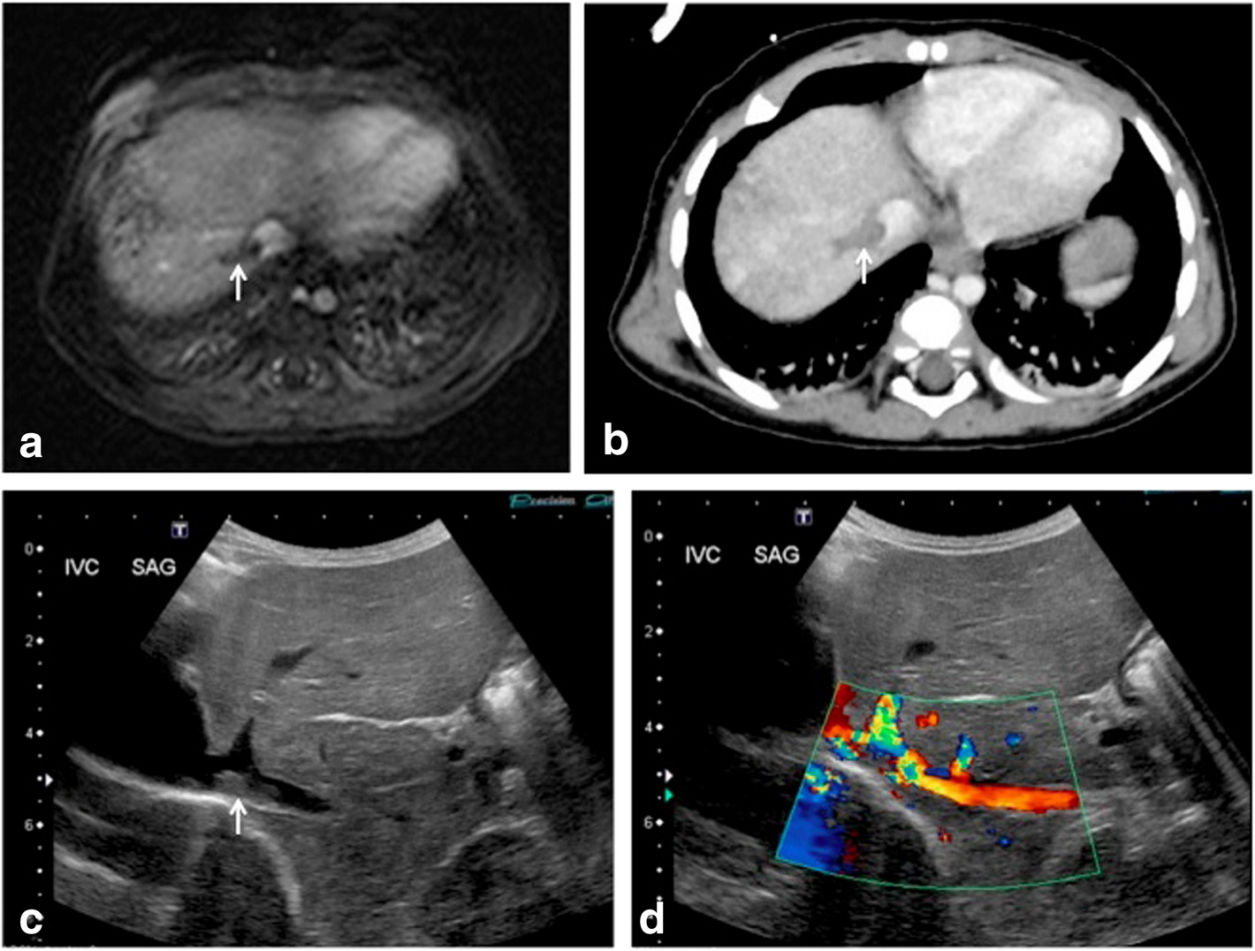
Metastatic hepatoblastoma with inferior vena cava (IVC) and right hepatic vein thrombus (*white arrows*) in a 2 year old boy. After administration of gadobenate dimeglumine, the (a) arterial phase T1-weighted fat-saturated imaging demonstrates a filling defect in the affected vessels. Thrombosis was subsequently confirmed and identified on (b) portal venous phase post-contrast imaging on CT and (c), (d) ultrasound imaging of the IVC with and without colour doppler overlay respectively
